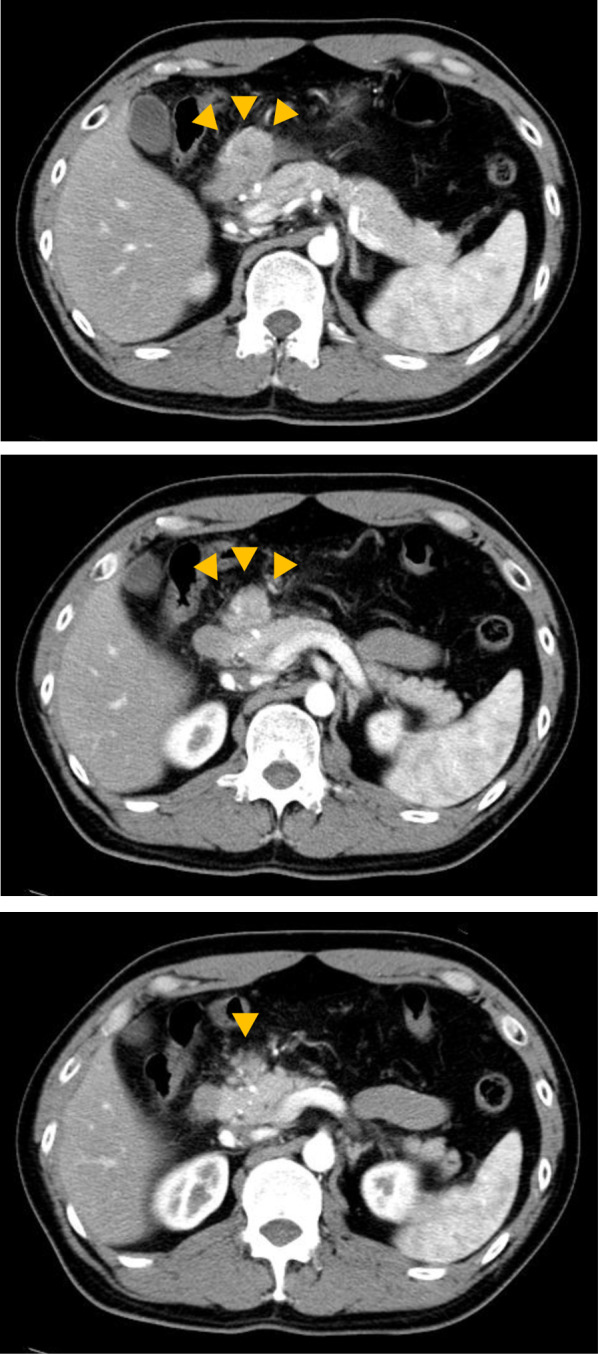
Findings of abdominal computed tomography. CT revealed a 25-mm-large mass lesion with contrast effect from the anterior wall of the gastric pylorus to the duodenum (yellow arrow). From the CT image, it is difficult to distinguish the continuity between the tumor and the pancreatic head, which looks more like a tumor. *CT* computed tomography
